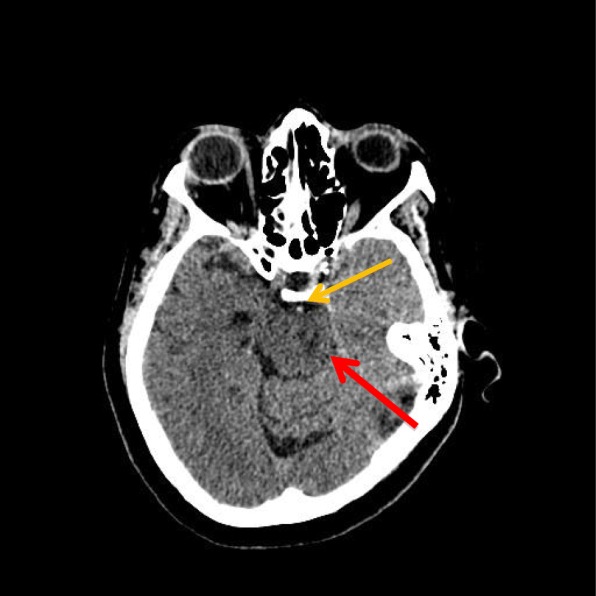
Axial computed tomography of the patient’s head without contrast from February 7, 2015. Image at the level of the brainstem demonstrates a hyperdense basilar artery (*orange arrow*), which in the appropriate clinical setting is concerning for occlusion of the basilar artery due to thrombosis. Also, note that the pons (*red arrow*) is slightly decreased in attenuation compared with the surrounding brain parenchyma, concerning for ischemia. This finding is slightly more prominent on the left side
